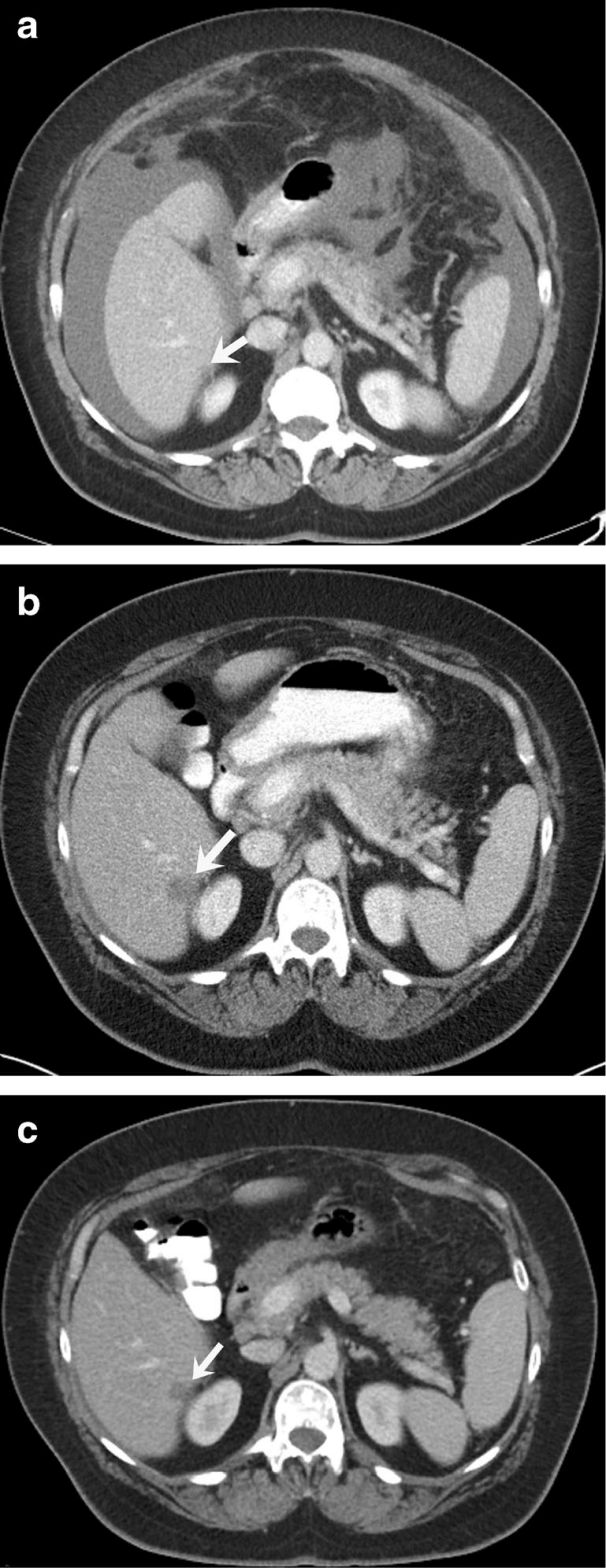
58-year-old woman with estrogen receptor-positive metastatic breast cancer treated with trastuzumab. a Axial contrast-enhanced CT image of the abdomen demonstrates a subcentimeter hypodense lesion in the liver (*arrow*) and ascites. b Follow-up scan after 3 months of treatment shows increase in the size of the liver metastasis with concurrent decrease in the ascites. Patient continued therapy due to decrease in tumour markers. c Repeat scan 2 months later shows decreased size of liver metastasis. The transient increase in the size of the metastasis on the interim scan (b) was due to decreased enhancement of the lesion suggestive of pseudoprogression
